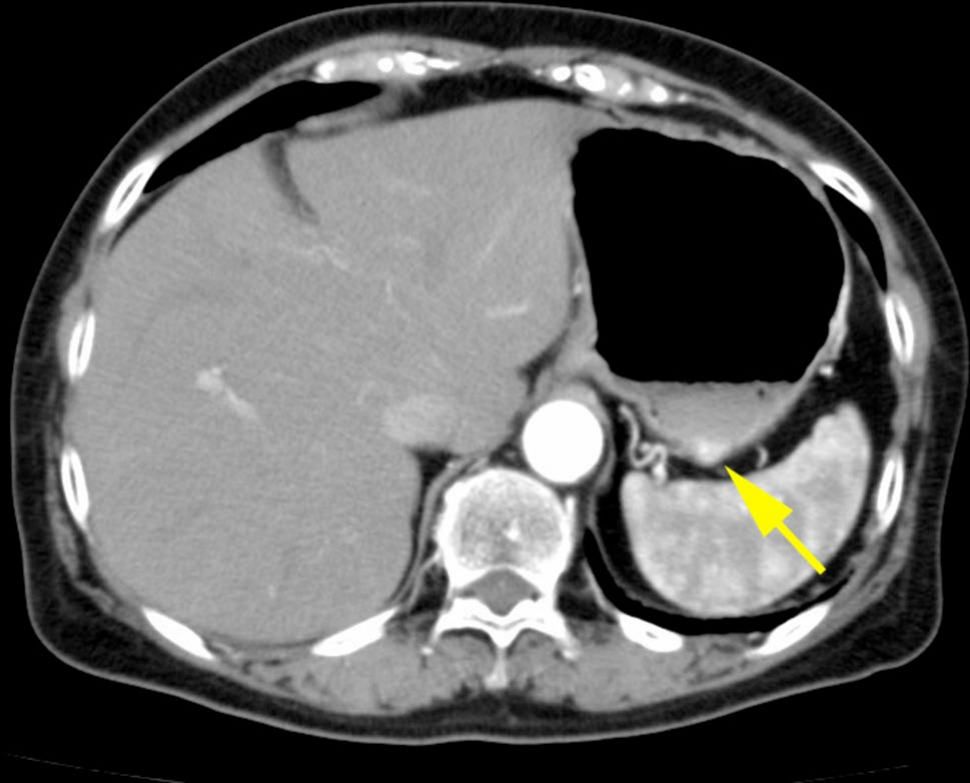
Contrast-enhanced computed tomography imaging. A low-attenuated tumor on the greater curvature of the stomach, measuring 16-mm diameter, with slow enhancement
